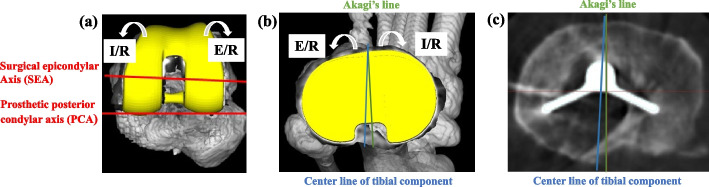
The postoperative three-dimensional computed tomography image (3DCT) image are shown. a Femoral component rotational angle. Axial 3DCT image of the left femur. The surgical epicondylar axis (SEA) connects the lowest point of the medial epicondyle to the midpoint of the lateral epicondyle. The prosthetic posterior condylar axis (PCA) connects the medial and lateral prosthetic posterior condylar surfaces. The femoral component rotational angle was defined as the angle between the SEA and the PCA. b, C Tibial component rotational angle. Axial 3DCT image of the left tibia. Akagi’s line connects the center of the posterior cruciate ligament and the medial border of the tibial tuberosity. The tibial component rotational angle was defined as the angle between the centerline of the tibial component and Akagi’s line. A positive value represents external rotation, and a negative value represents internal rotation of the femoral and tibial components. The absolute value of the angular divergence of the femoral component relative to the tibial component is defined as the rotational mismatch [27]. E/R; external rotation, I/R; internal rotation
